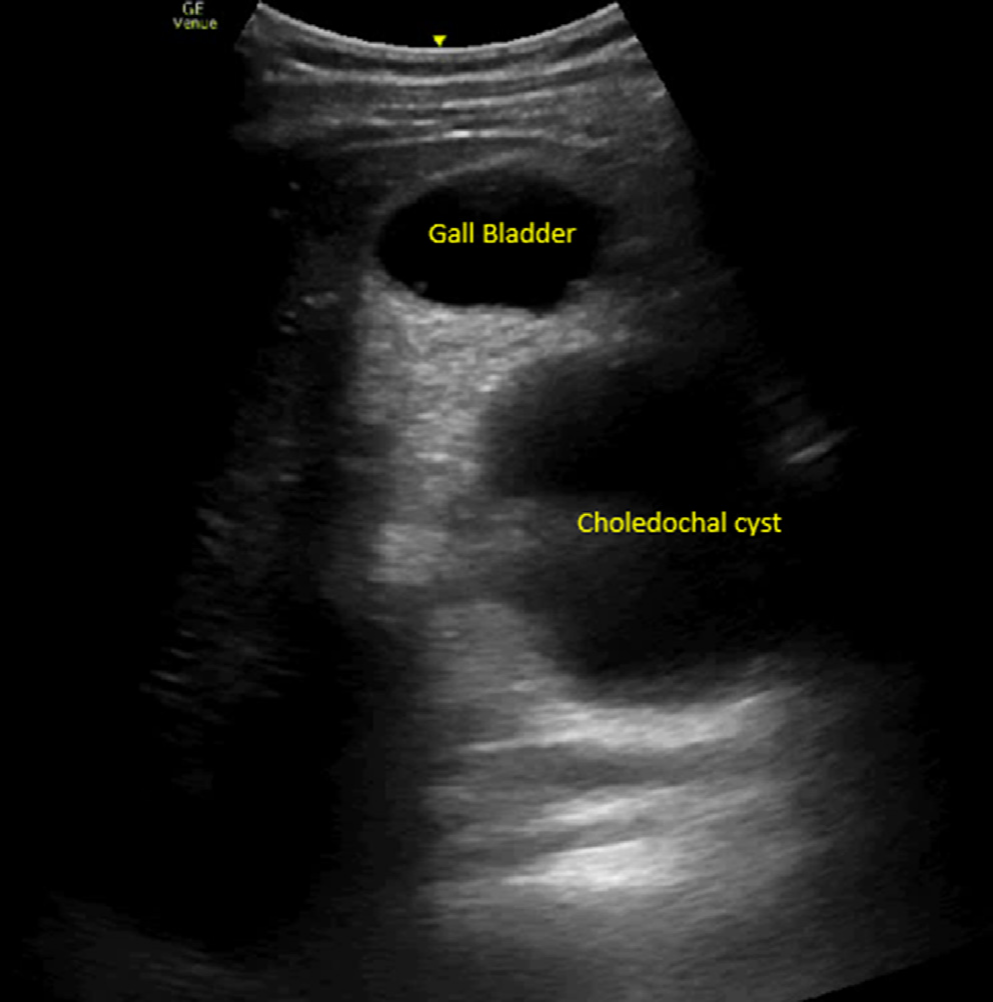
Point-of-care ultrasound demonstrated a normal gall bladder and large choledochal cyst. The choledochal cyst is easily mistaken as part of the gall bladder in this view.
